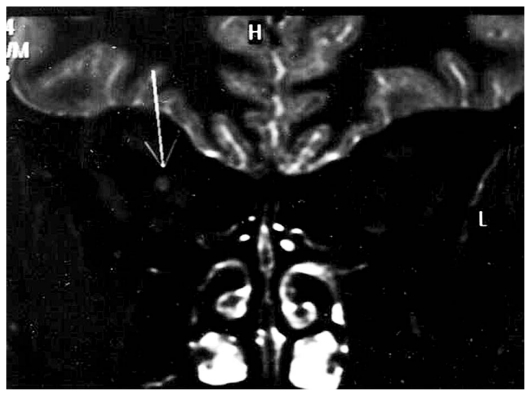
Lyme disease: MRI appearance of right optic nerve enhancement after administration of paramagnetic contrast (T1-weighted image).
